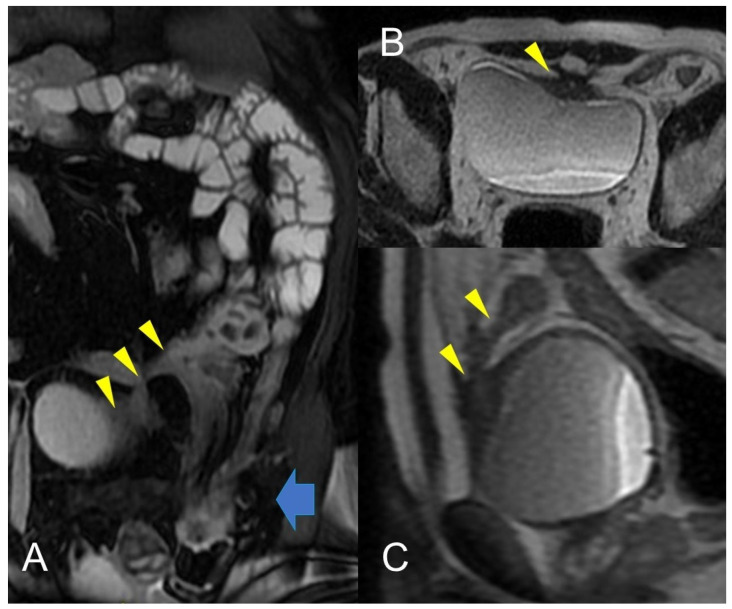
Crohn’s disease is a common cause of Enterovesical fistula formation. The enterovesical fistula represents an abnormal connection between the enteric lumen and the bladder. Coronal fast imaging employing steady-state acquisition (FIESTA) images (A) show a complex fistula with one arm consisting of an enterovesical fistula (yellow arrowheads). Axial (B) and sagittal (C) T2-weighted MRE image of the same patient shows enterovesical fistula. In (A), inguinal hernia indicated by the blue arrow with involvement of a pathological section of the small intestine.
